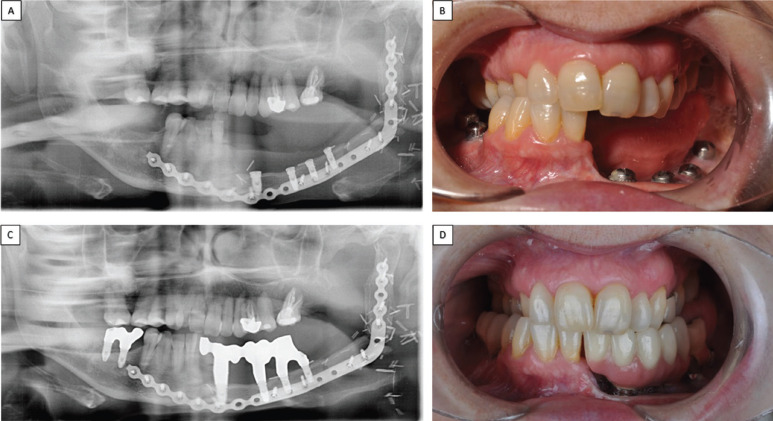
A,B. Orthopantomography and frontal photograph showing OII placement in the fibular free flap region bearing implants with corresponding healing abutments. C,D. Orthopantomography and photograph showing the patient with prosthesis placed in oral cavity.
